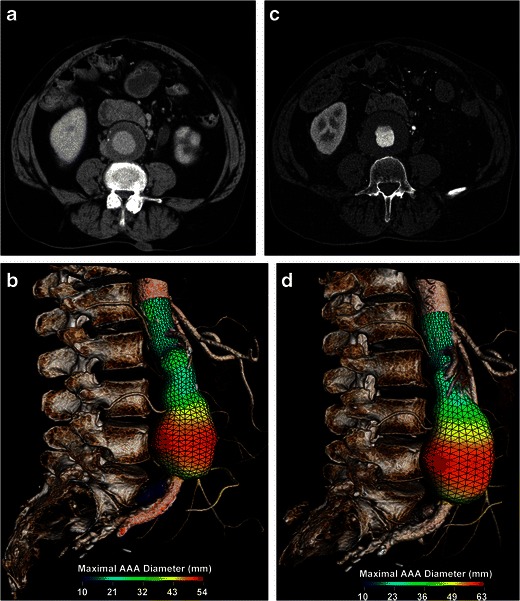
Rapid enlargement rate. a Axial enhanced CT and b 3D rendering image with a colour parametric map of a 71-year-old man shows a 5.4-cm AAA. c Axial enhanced CT and d 3D rendering image with a colour parametric map of the same patient performed a year later shows a 1 cm increase in diameter
